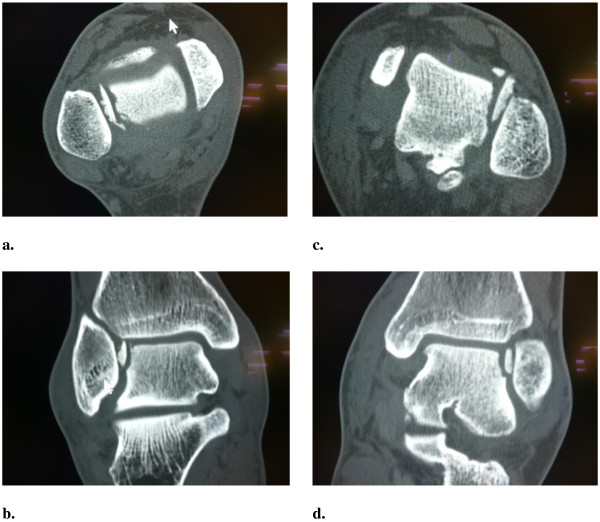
Case pair D, paired CT-scan images (a and c transverse plane, b and d coronal reconstructions) of the talus. Images a and b from the left board gunner, c and d from the board gunner on the right. Both occupants sustained an inverted osteochondral chip fracture of the lateral talus dome.
